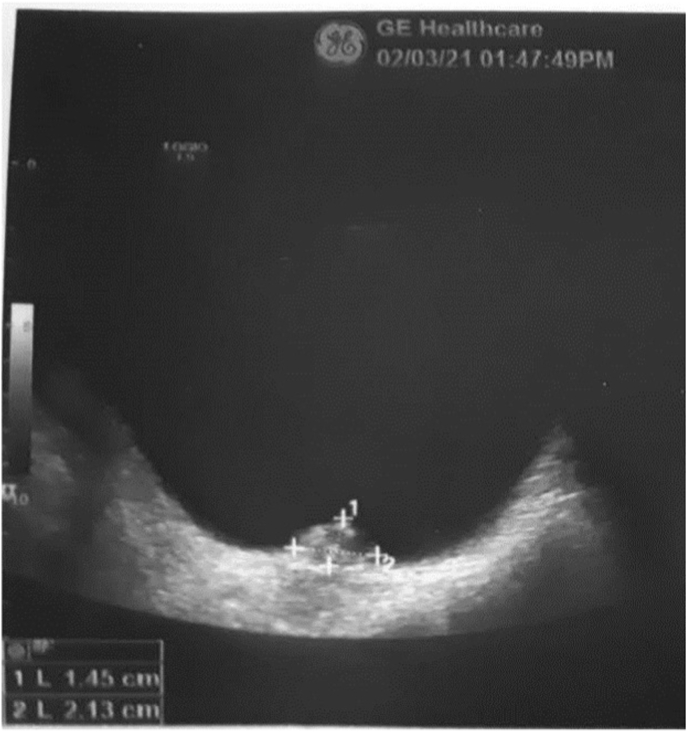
Ultrasound of the bladder showing a hyperechoic mass (14.5 mm × 21.3 mm) with multiple echogenic foci.
